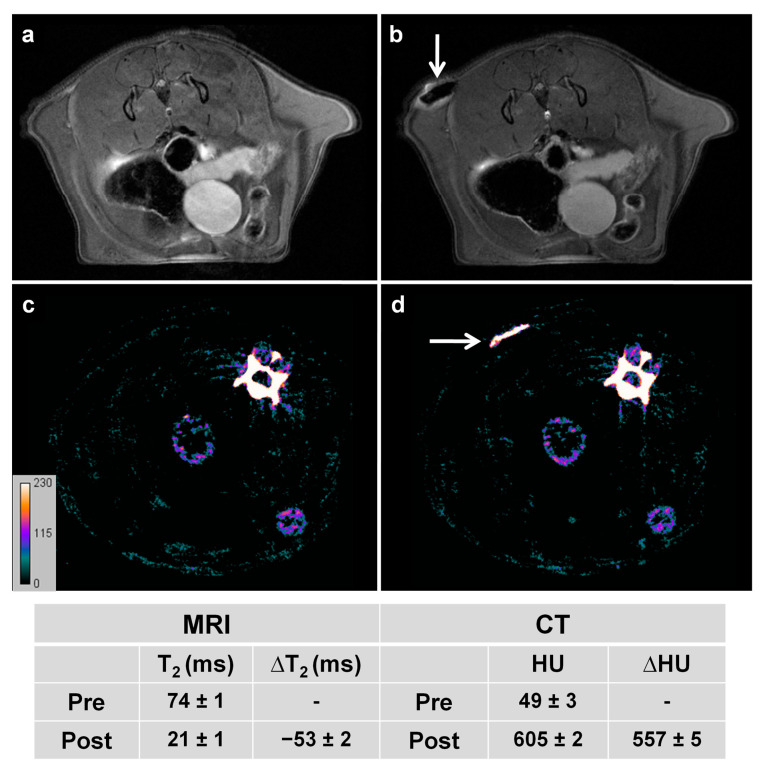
Representative in vivo images before (a,c) and after (b,d) subcutaneous administration of Fe@Au NPs. Top: T_2_-weighted images; bottom: CT images. Arrows indicate NPs location. The table collects the corresponding ΔT_2_ and ΔHU values.
